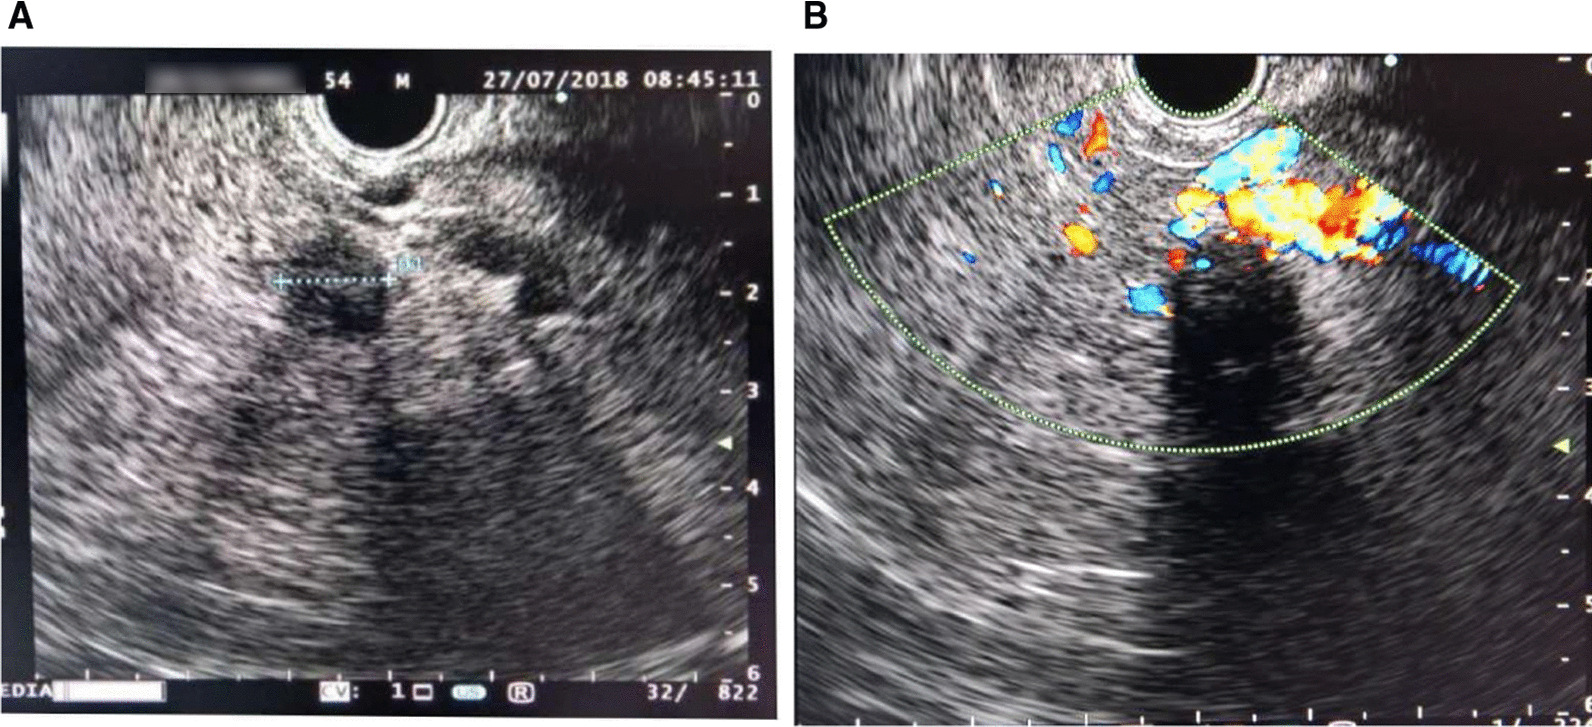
A Endoscopic ultrasound of our patient showing a 12 × 12 mm mass lesion of the pancreatic tail; B endoscopic ultrasound showing a mass lesion of the pancreatic tail with overlying splenic arteries (risk of perforation on fine-needle biopsy)
